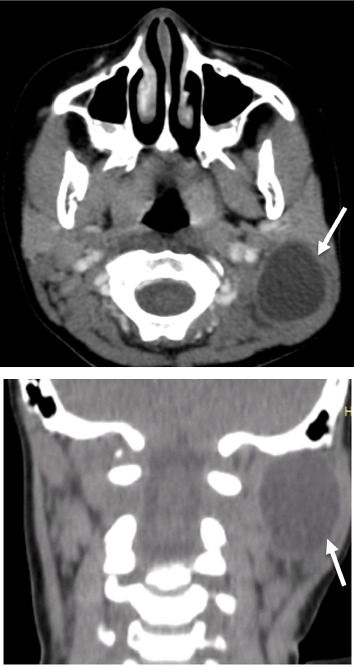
Preoperative axial and coronal CT scan images of the neck showing a left posterior neck unilocular cystic lesion (arrow).
